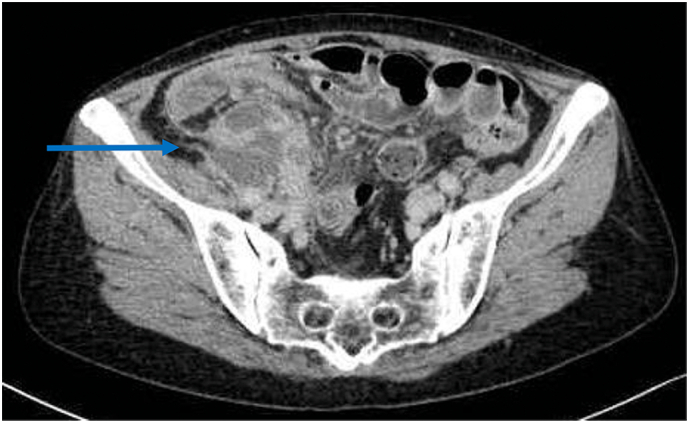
Computed tomography revealed an abscess around the cecum (blue arrow). (For interpretation of the references to colour in this figure legend, the reader is referred to the web version of this article.)
